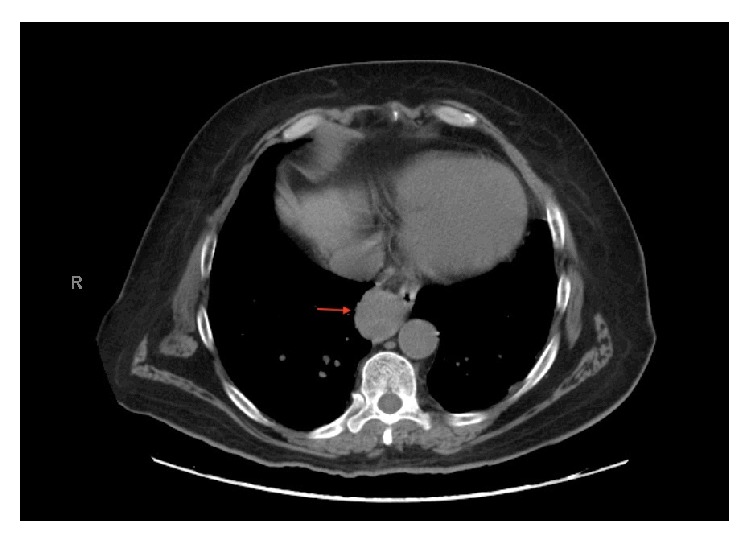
CT scan showing a 3.5 cm azygoesophageal recess mass closely approximating the esophagus.
